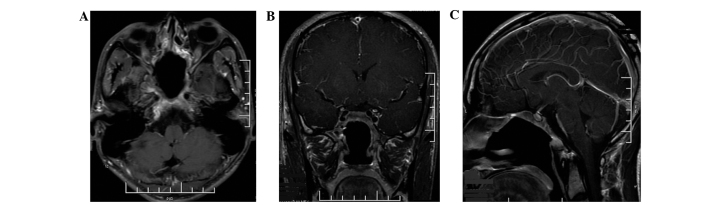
(A) Axial, (B) coronal and (C) sagittal section of postoperative T1-weighted magnetic resonance imaging with contrast revealing no residual or recurrent tumor.
